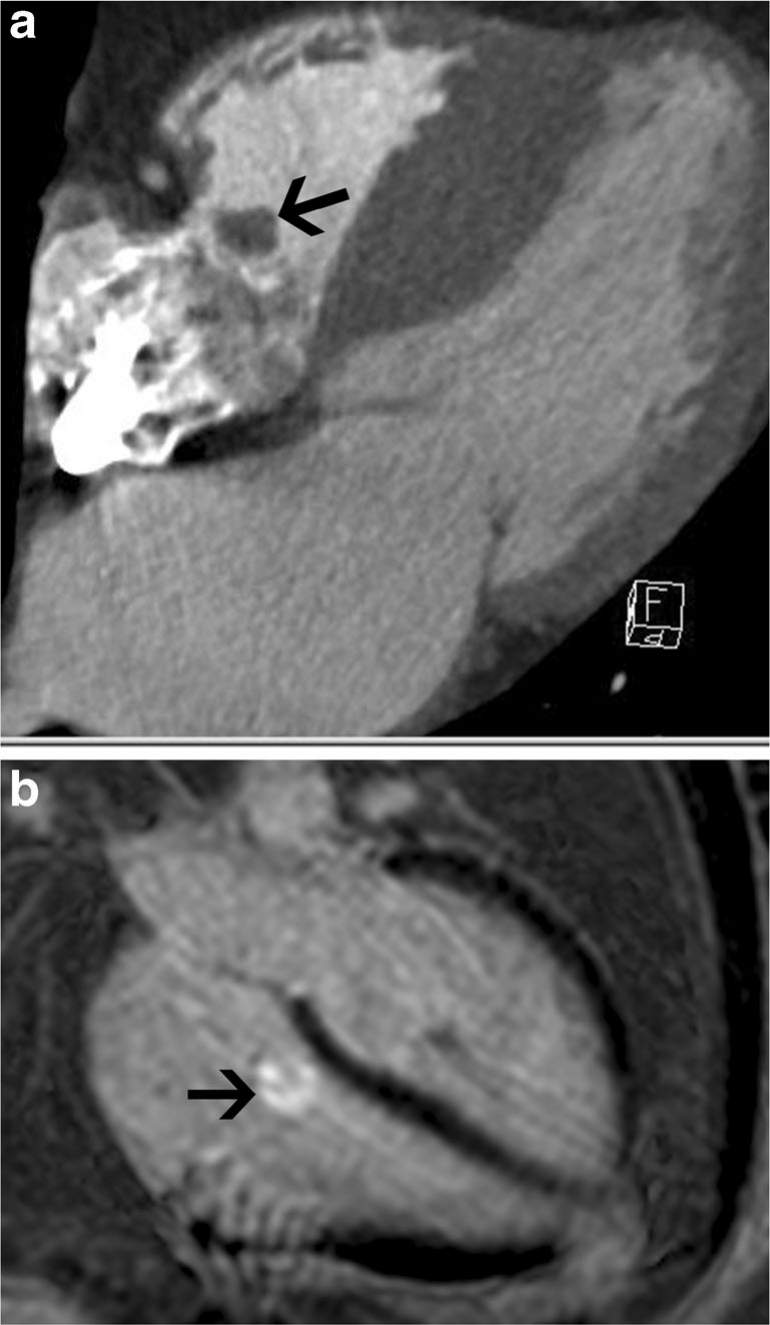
Fibroelastoma. (a) Four-chamber CT image shows a small, well-defined mass attached to the septal leaflet of the tricuspid valve (*arrow*), consistent with a fibroelastoma. (b) Four-chamber delayed-enhancement image in another patient shows heterogeneous enhancement of the tricuspid valve mass, consistent with a fibroelastoma
